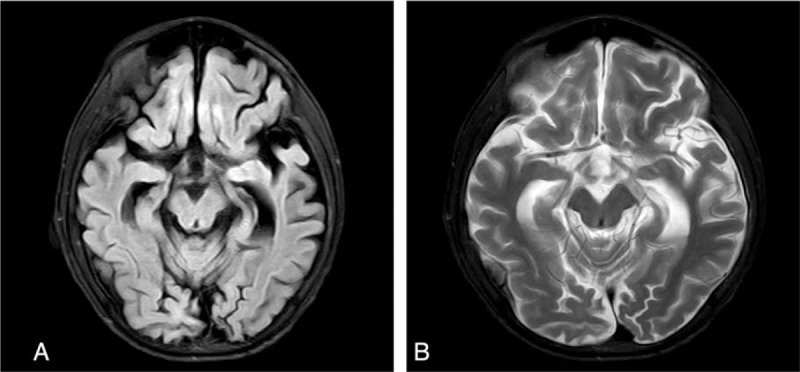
Cerebral magnetic resonance imaging (MRI) on November 28, 2017. Cerebral magnetic resonance imaging examinations on November 28, 2017 showed atrophic changes of the cerebrum accompanied by basal ganglia atrophy (A: FLAIR;B: T_2_WI).
